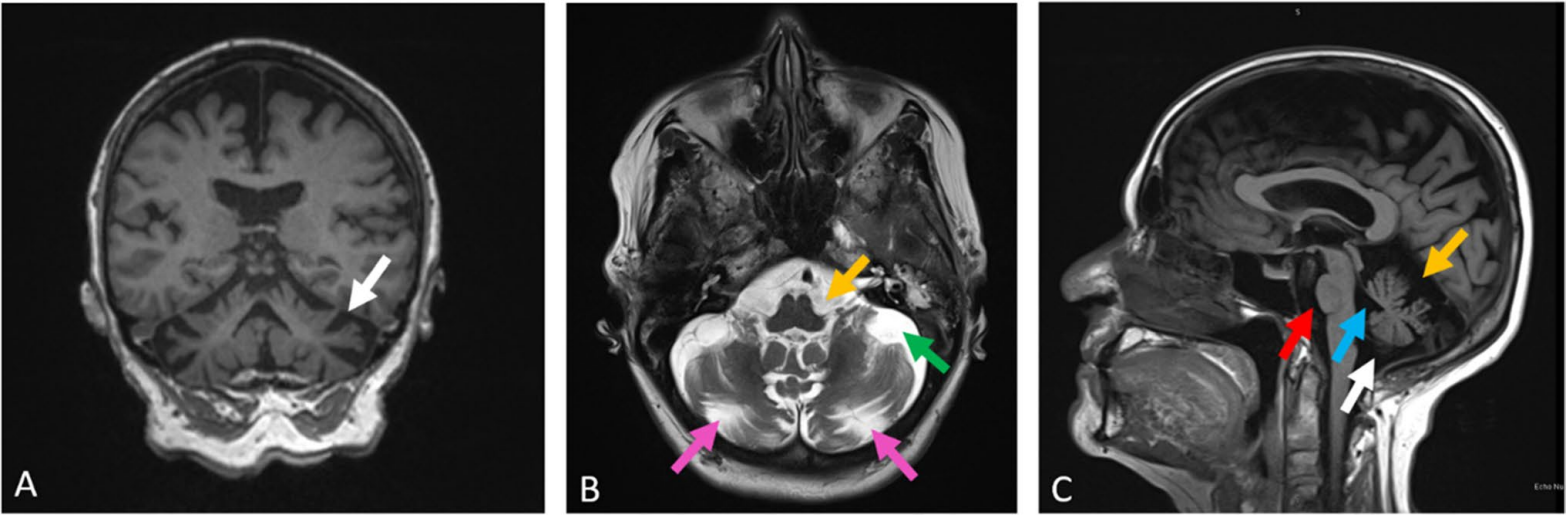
Brain MRI of III-3 showing severe cerebellar atrophy with moderate pontine and medullary atrophy. A T1 weighted coronal MRI showing generalised atrophy of cerebellum (white arrow); B T2 weighted axial MRI showing enlargement of the pontine cistern (yellow arrow), cerebellomedullary cistern (green arrow), and ex-vacuo prominence of the horizontal fissure of the cerebellum (pink arrows); C T1 weighted midline sagittal MRI showing enlarged pontine cistern (red arrow), fourth ventricle (cyan arrow), superior cistern (yellow arrow), and cerebellomedullary cistern (white arrow) with sparing of cerebrum
